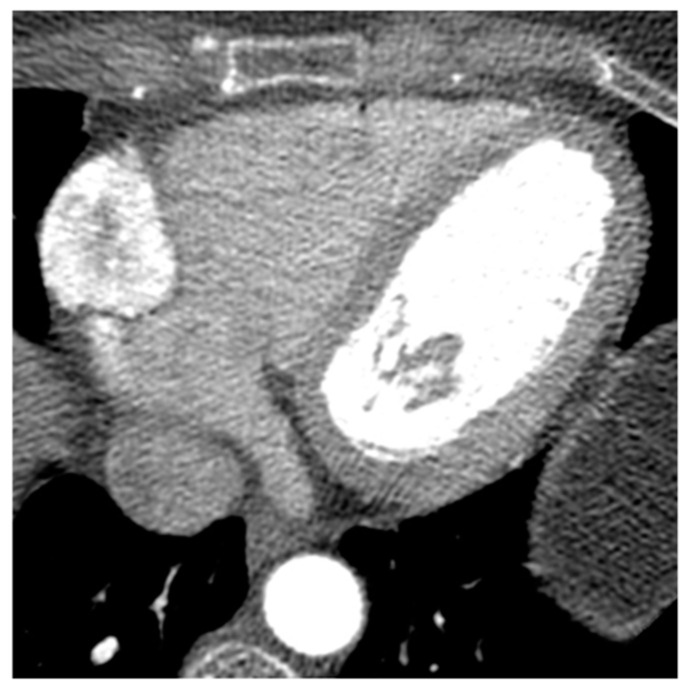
A right atrioventricular groove paraganglioma with predominant peripheral enhancement due to central necrosis on arterial phase CT image.
